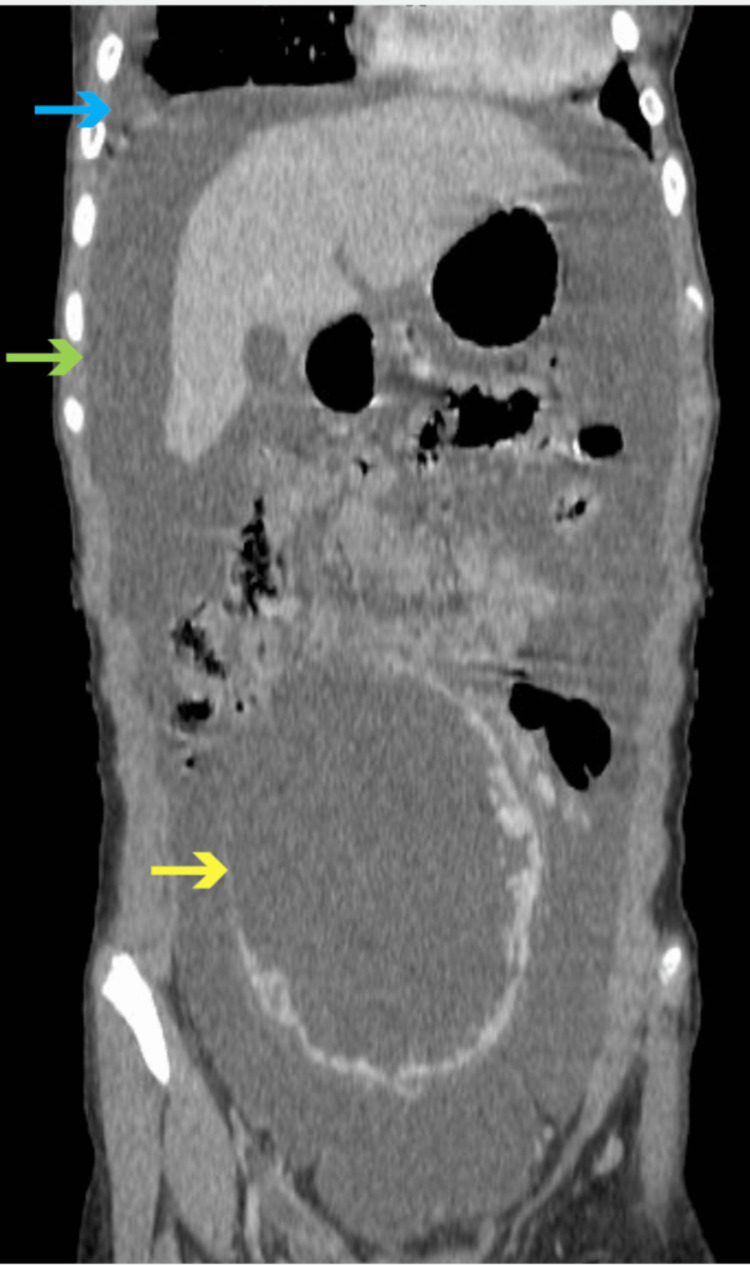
Computed tomography. Mild-to-moderate right pleural effusion (blue arrow) and a large heterogeneously enhancing complex cystic mass arising from the left ovary measuring 14.8 × 12.5 × 6 cm (yellow arrow), along with large ascites (green arrow) were noted.
